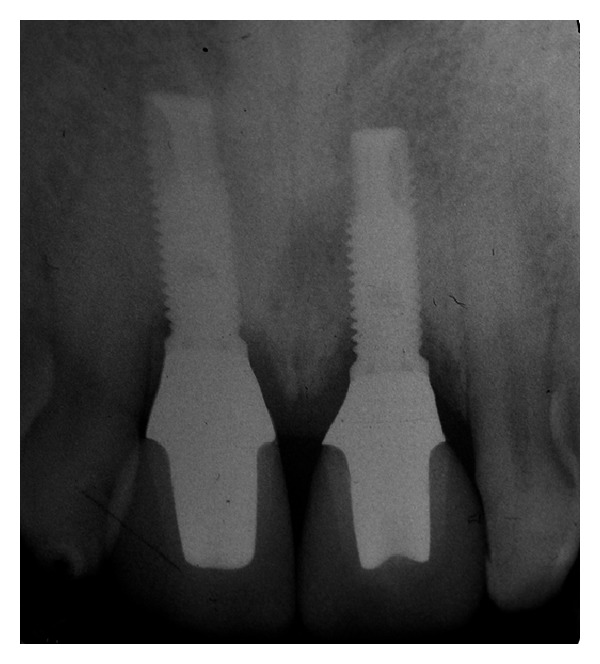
Periapical radiograph after 5 years which showed osseointegration of the implants with only a minimal loss of bone height according to the Albrektsson criteria.
